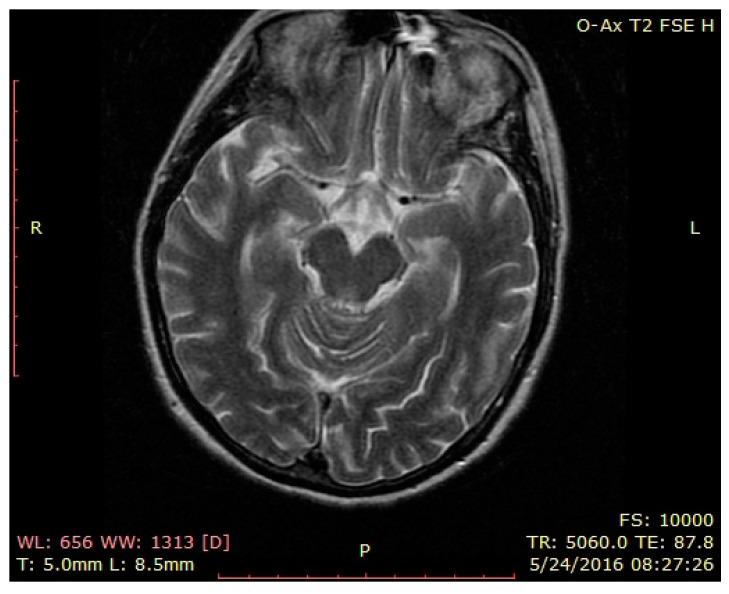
Brain MRI—normal findings (T2 weighted brain scan), axial temporal image.
